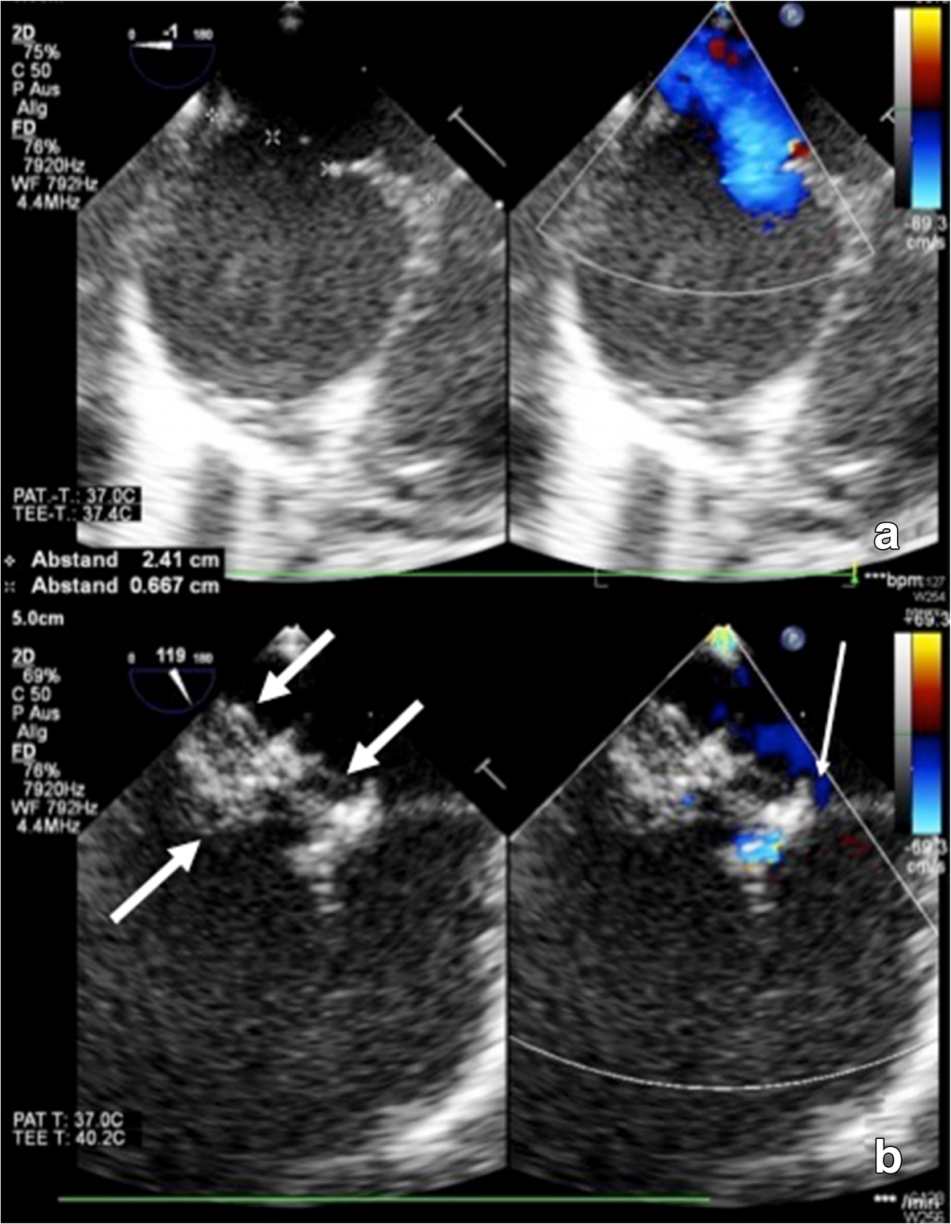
Transoesophageal echocardiography during interventional ASD closure. Echocardiographic measurement of the larger ASD prior to interventional closure (a). Echocardiographic image after successful implantation of a GCSO (bold arrows) into the larger ASD. Incomplete coverage of the smaller ASD resulted in trivial residual shunting (slim arrow) (b)
